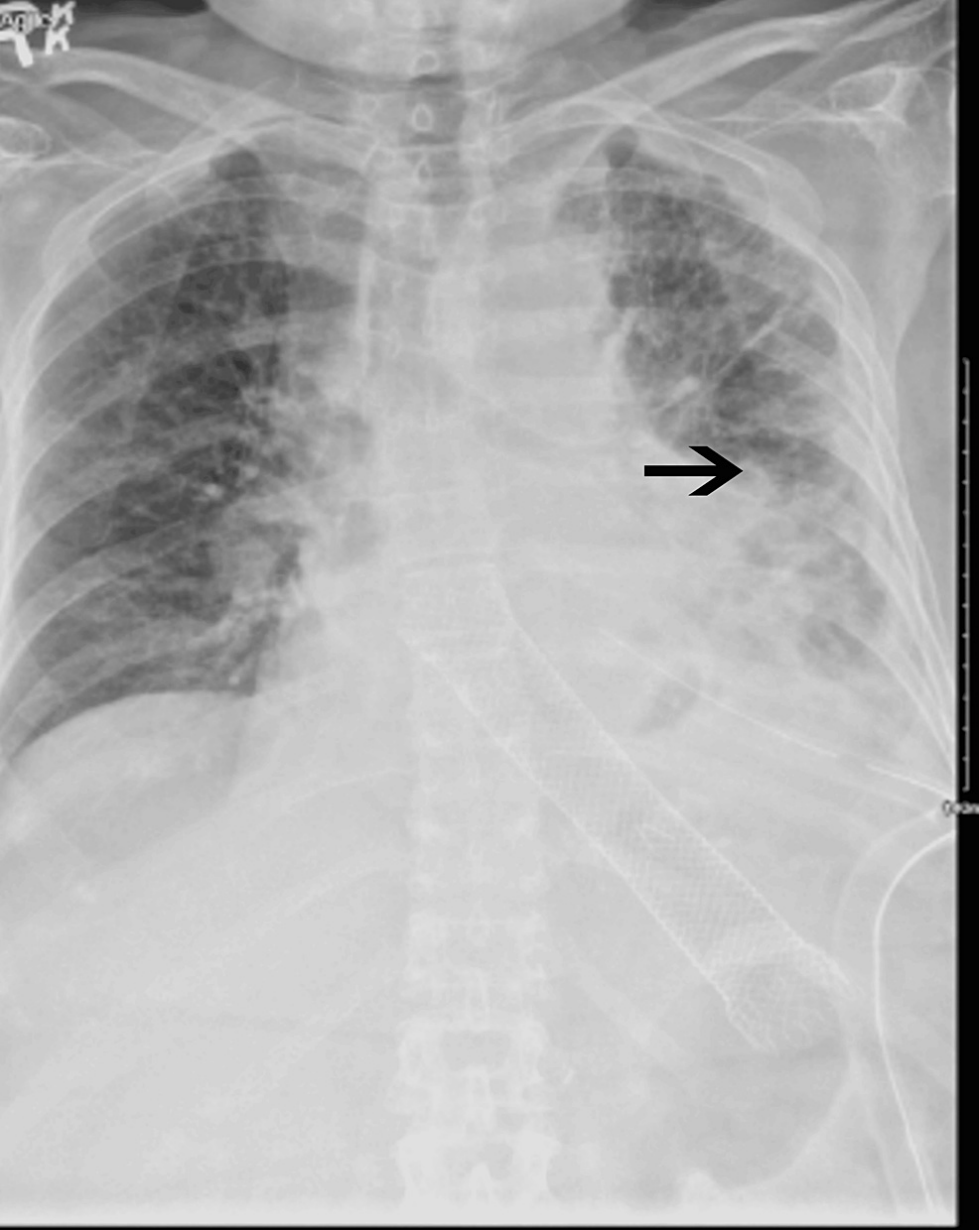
Repeat chest X-ray post surgery showing improvement in left-sided pleural effusion.
